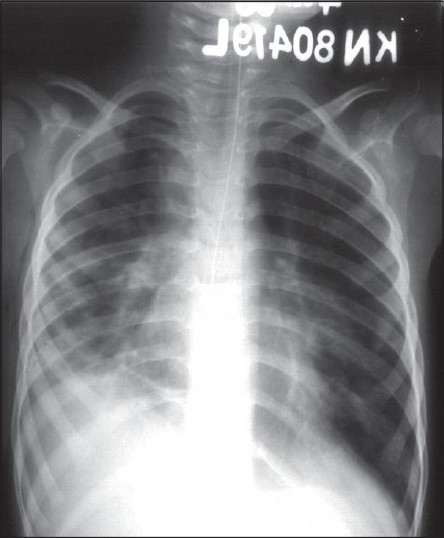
Radiograph showing ventilator-associated pneumonia in right lower zone.
